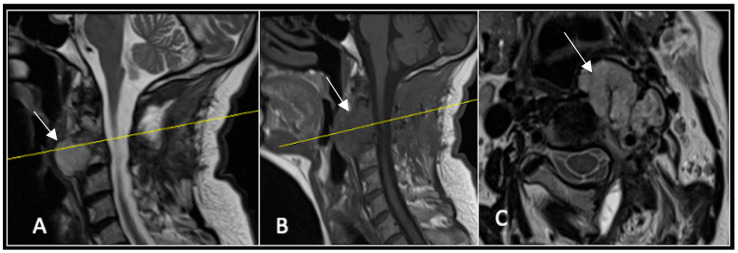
MRI demonstrating a left-sided posterior approach to the cervical spine with significant reduction in residual gross tumour volume (arrow) and circumferential decompression of the spinal cord. The yellow line on the sagittal images denotes the level corresponding to the axial section. (A) T2-weighted sagittal; (B) T1-weighted sagittal; (C) T2-weighted axial.
